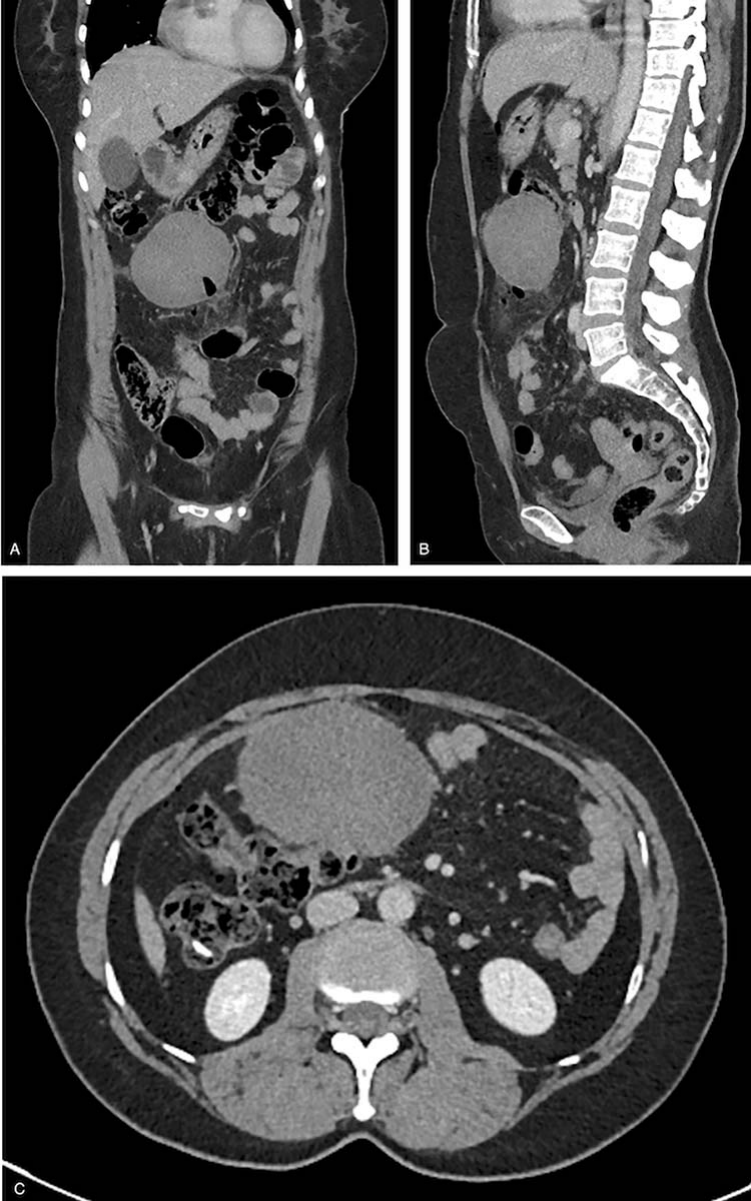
CT of the abdomen and pelvis preoperatively. (A) Coronal, (B) sagittal and (C) axial. The location and the size of the tumour are obvious. Also, the locules of gas are seen in (A) and (B).
